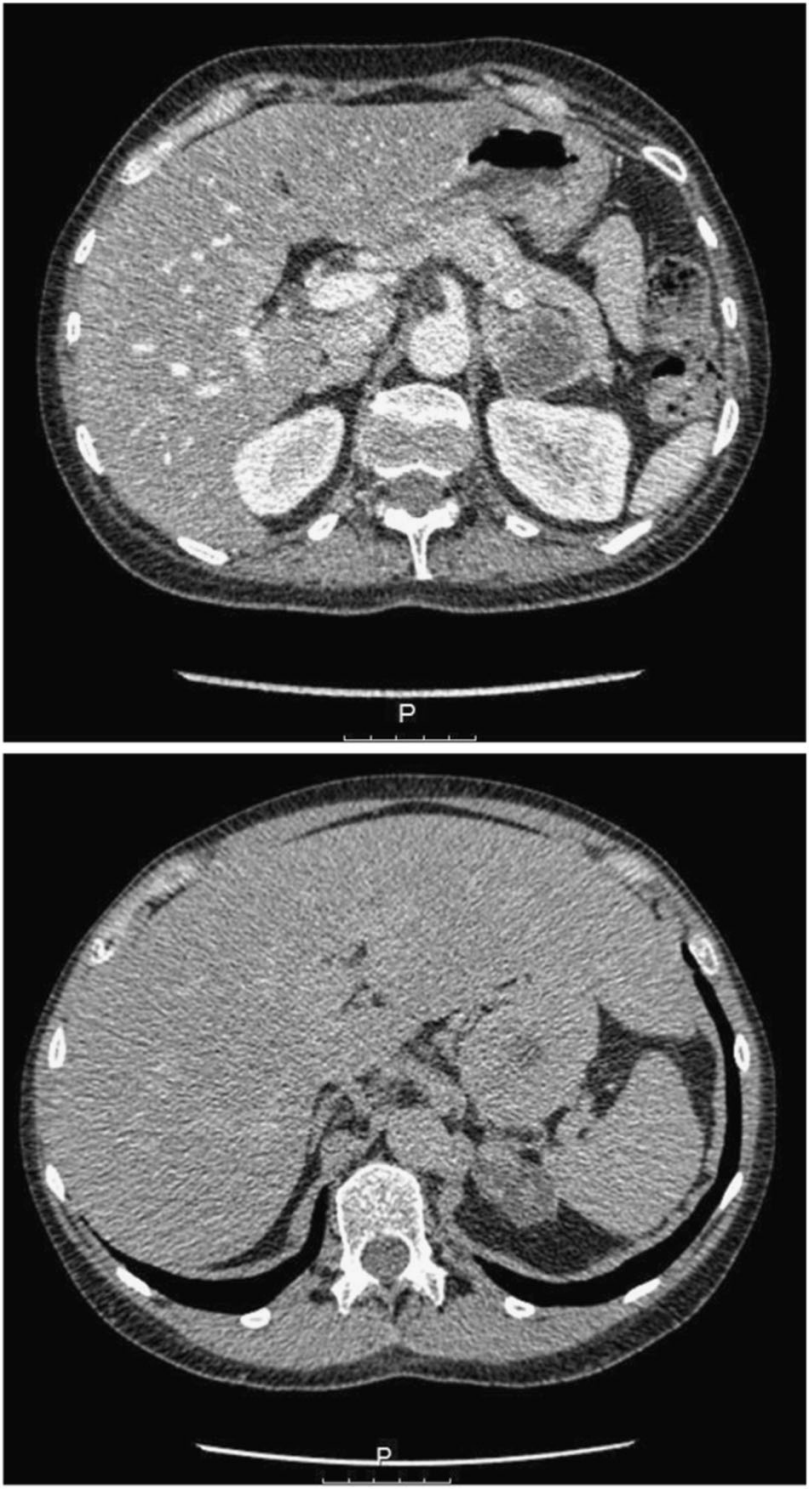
CT with contrast from Case 1 demonstrating a left-sided 3.5 × 4.5 cm large adrenal tumor with peripheral contrast enhancement and septa-like structures in addition to bilateral adrenal hyperplasia (*upper panel*). CT without contrast from Case 2 showing a left-sided 3.5 × 4.5 × 5 cm large heterogeneous adrenal mass (*lower panel*)
